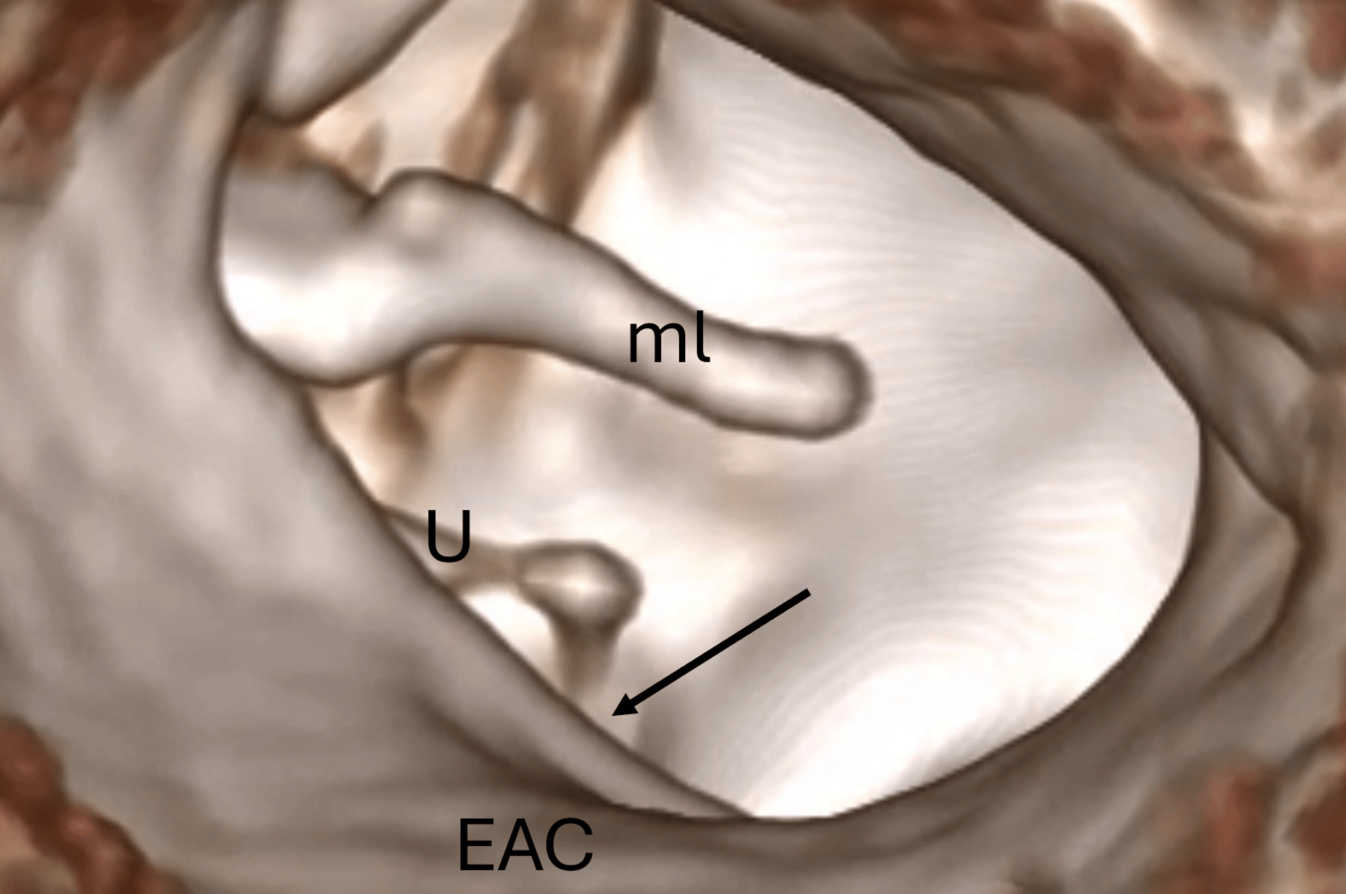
3D virtual endoscopic view based on CT scan CT: computed tomography; EAC: external auditory canal; ml: malleus; U: uncus
